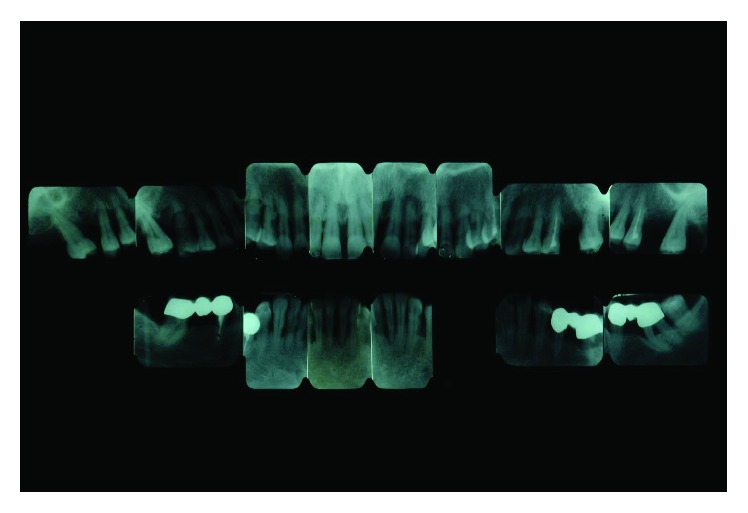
Initial case: full-mouth intraoral radiographic exam (May 2000).
